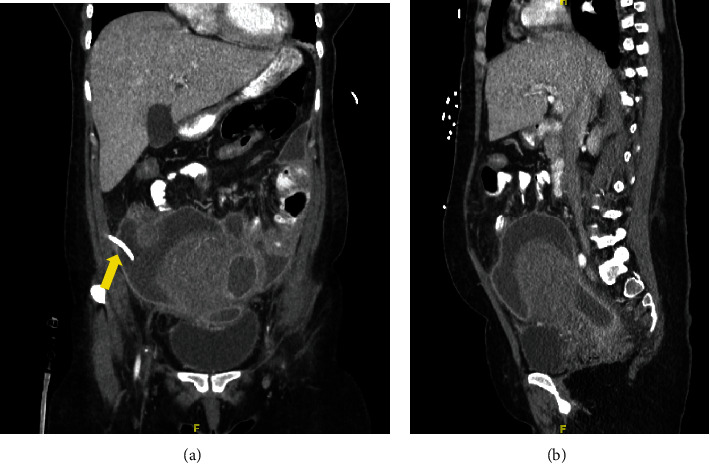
Coronal (a) and sagittal (b) views of the repeat CT abdomen and pelvis with IV and PO contrast performed 3 days after IR drainage and JP drain (arrow) placement still demonstrating multiple, large loculated fluid-filled collections.
